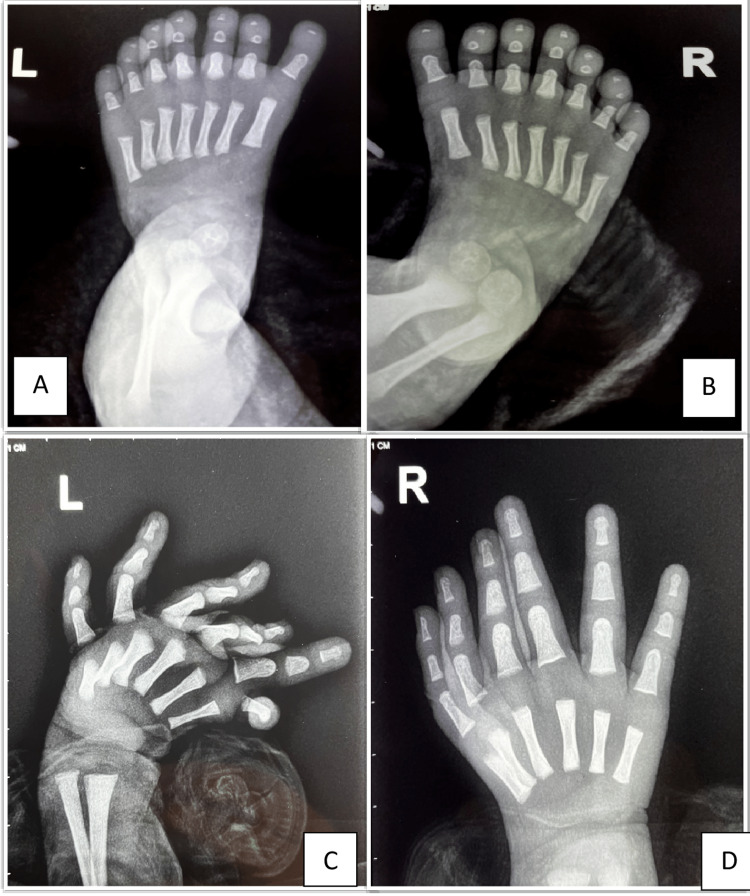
X-ray of both hands and feet showing distribution and number of digits Written consent from parents has been taken to publish their baby's pictures for medical education purposes.
